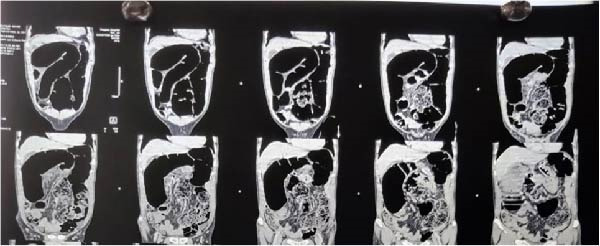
Coronal CT scan demonstrating dilated colonic segments and whirl‐like twisting of mesenteric vessels, suggestive of synchronous volvulus.
